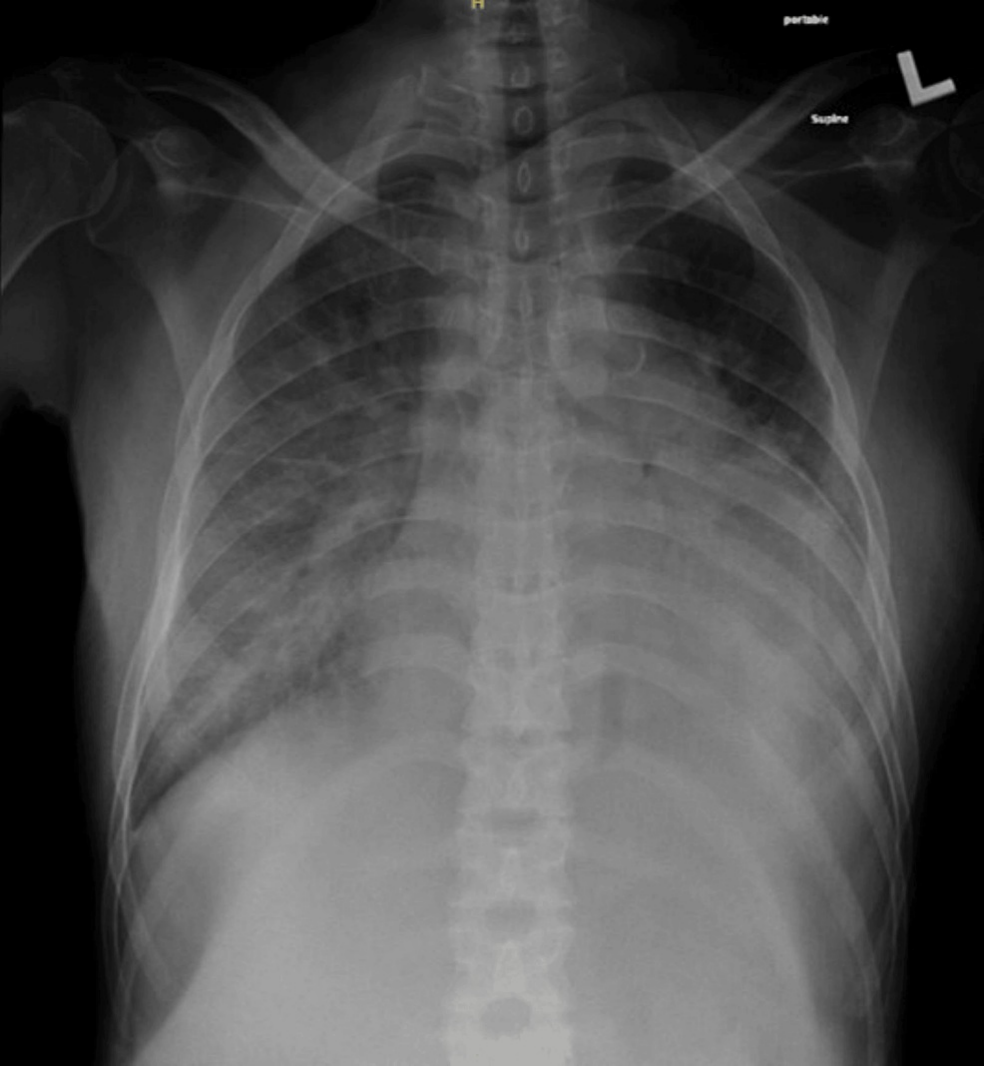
Anteroposterior chest X-ray showing flush pulmonary edema.
